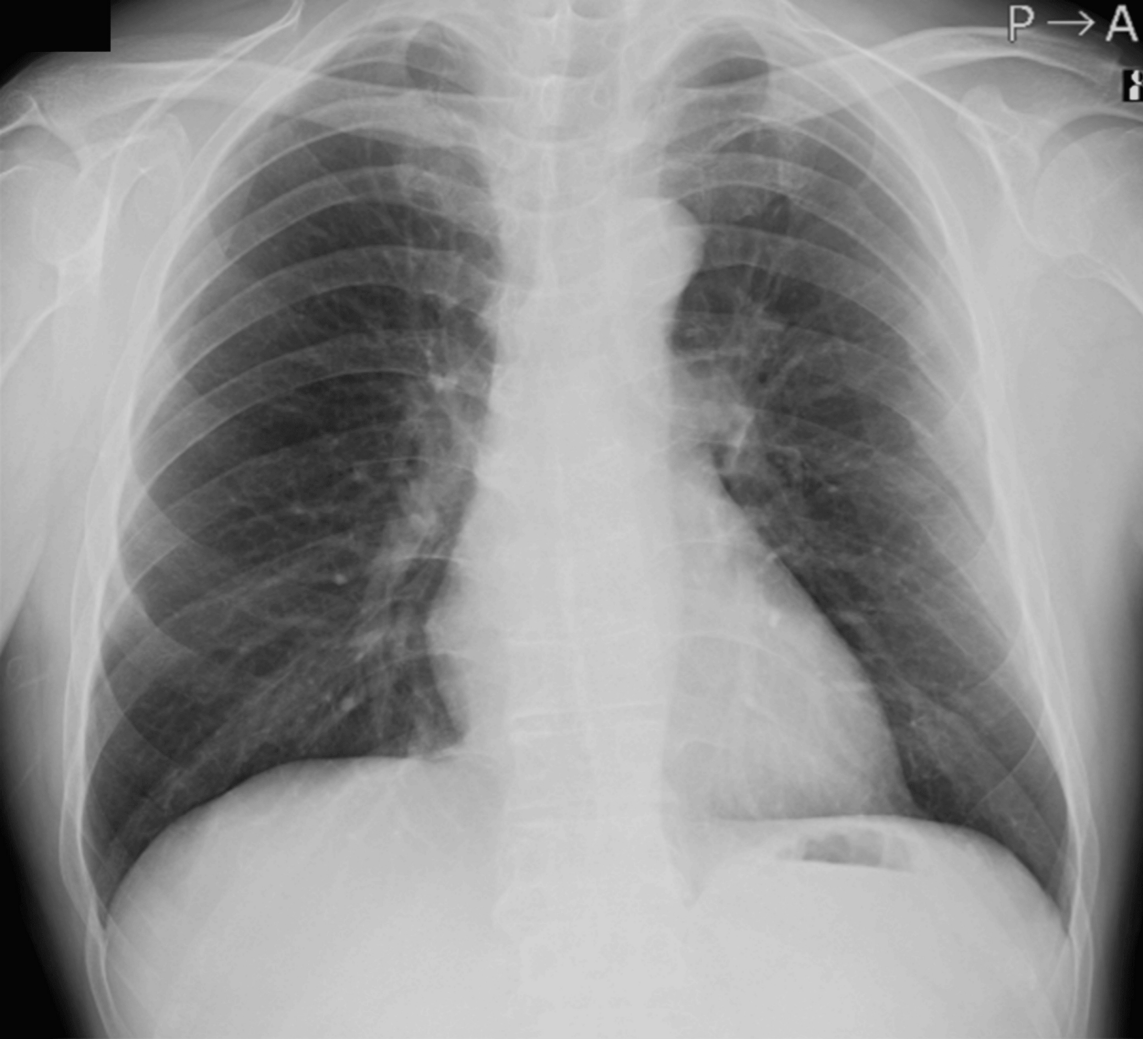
Chest radiograph No evidence of cardiomegaly, pleural effusion, or pulmonary congestion.
